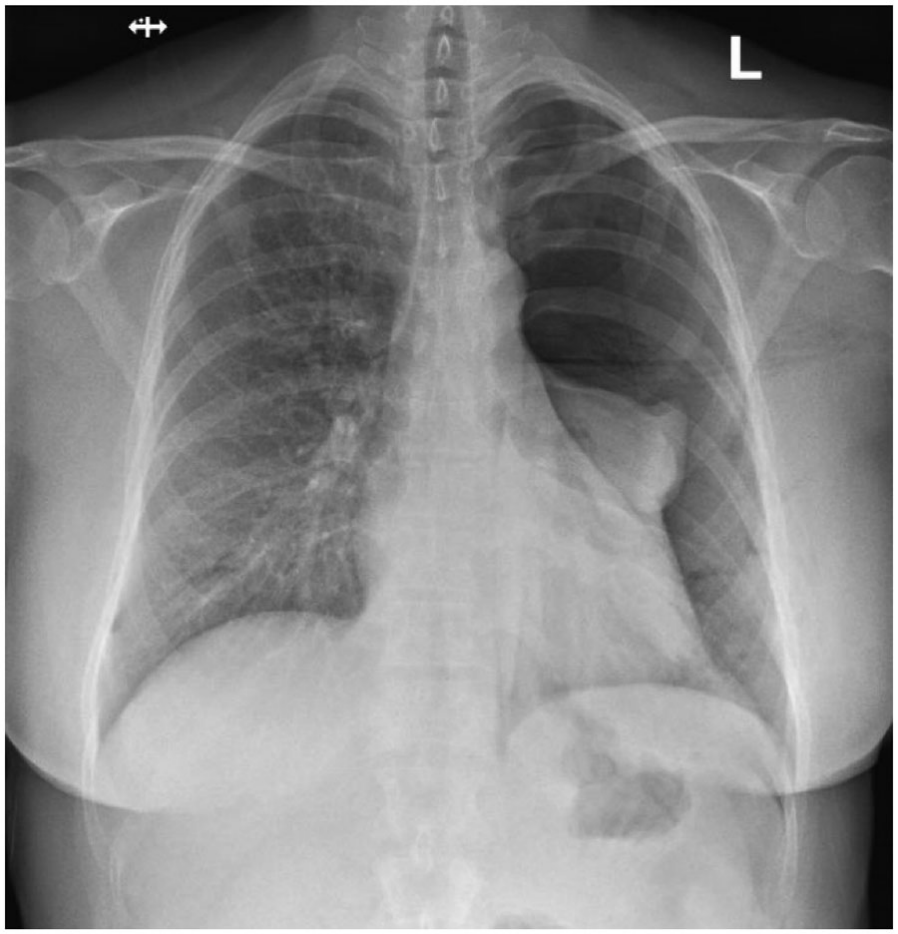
Postoperative chest X-ray image showing pneumothorax on the left side.
